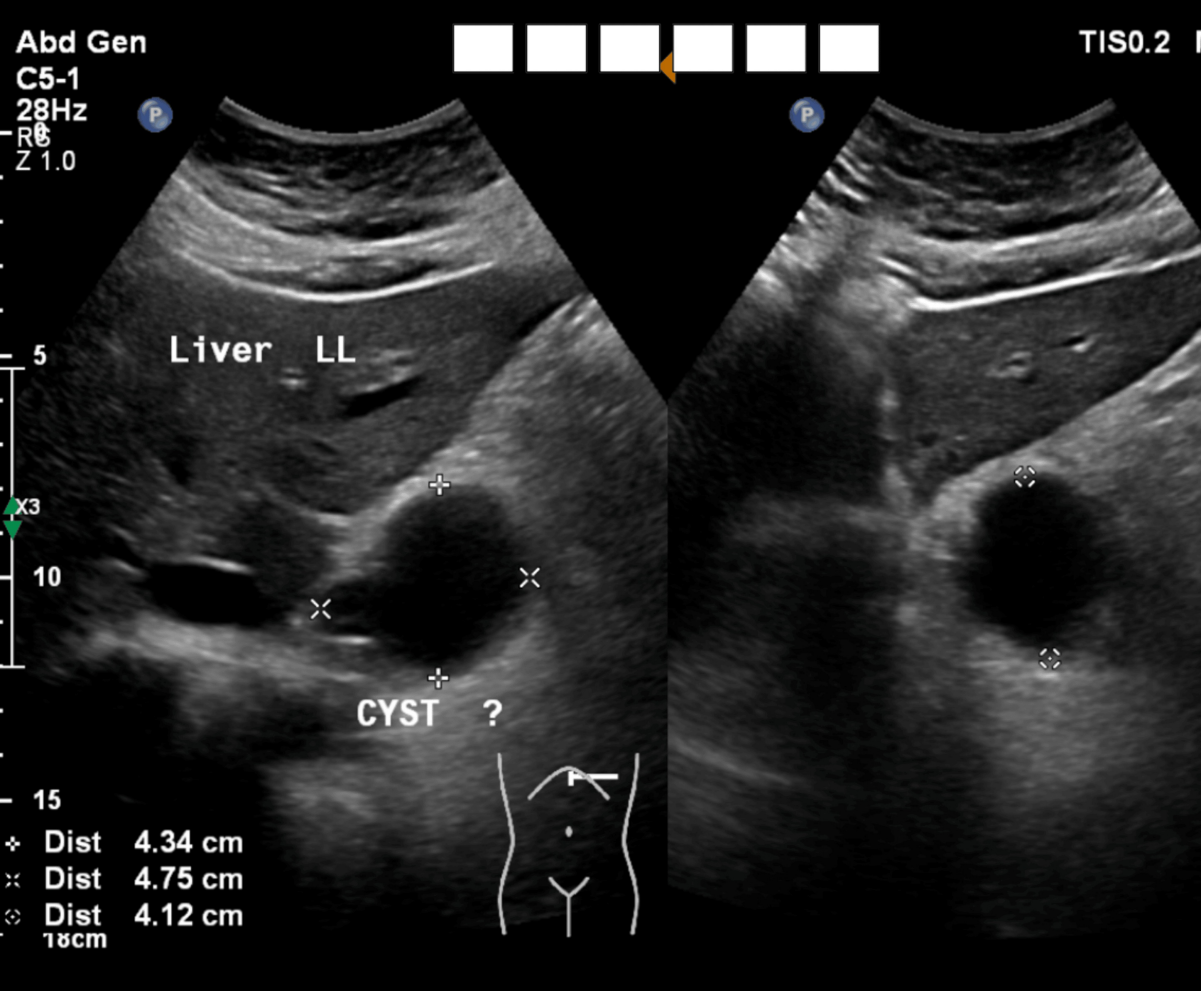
Ultrasonography findings A rounded anechoic cystic lesion was identified in the midline upper abdomen, lateral to the pancreatic head, and in the subhepatic region. The lesion measured 4.3 × 4.7 × 4.1 cm and demonstrated no internal vascularity or calcification. These findings were considered most consistent with a pancreatic pseudocyst
